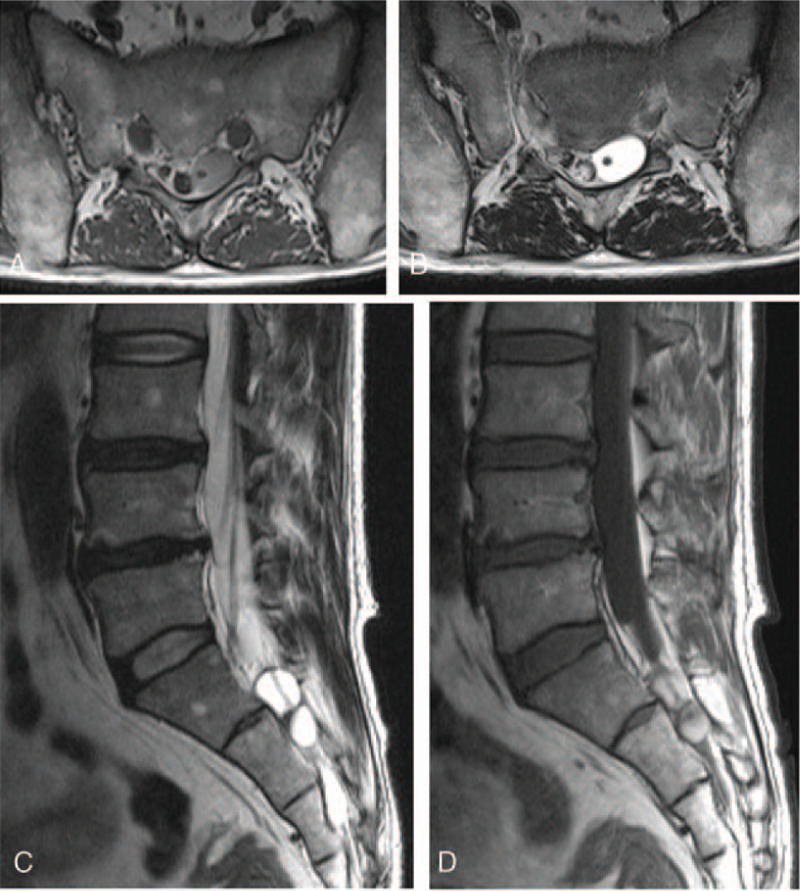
Non-enhanced T1-weighted (A) and T2-weighted (B) axial MRI showed the perineural cyst located to the left side and encompassing the nerve root. The left nerve root was smaller than the right S2 root because it was compressed by increased intracystic pressure. (C) T2-weighted sagittal MRI revealed 2 separated perineural cysts with high signal intensity at S1 and S2 levels. There was a nerve root inside the cyst (arrow). (D) Non-enhanced T1-weighted sagittal MRI showed mixed high and low signal intensity with a fluid-fluid level within the cyst, suggestive of intracystic hemorrhage. MRI = magnetic resonance imaging.
